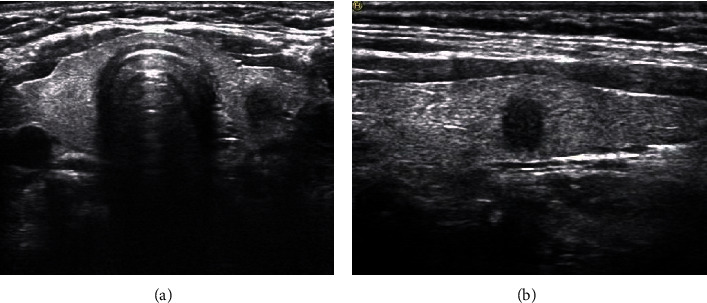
Ultrasonography of the nodules in papillary thyroid carcinoma (PTC) without central lymph node metastasis. The tumor is located in the middle of the left thyroid lobe, with a maximum diameter of 8 mm, solid and very hypoechoic, aspect ratio >1, and unclear margin. The American College of Radiology Thyroid Imaging Reporting and Data System (ACT TI-RADS) score was 8 points. (a) Transverse section; (b) longitudinal section.
